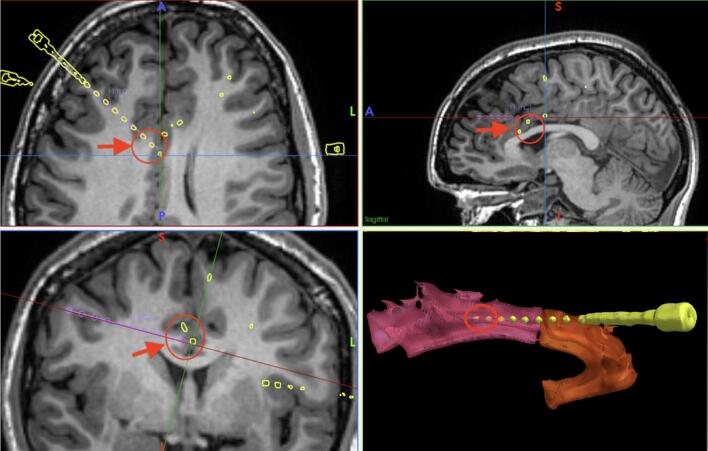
Stereo-EEG implantation plan showing the deep electrode position on MRI and the site of electrical cortical stimulation in the right mid-cingulate cortex. Contact sites encircled and indicated by the arrow: RmC1 (bottom) almost out in space below falx, RmC2 (middle) grey/white matter boundary, RmC3 (top) grey matter of middle cingulum cortex.
